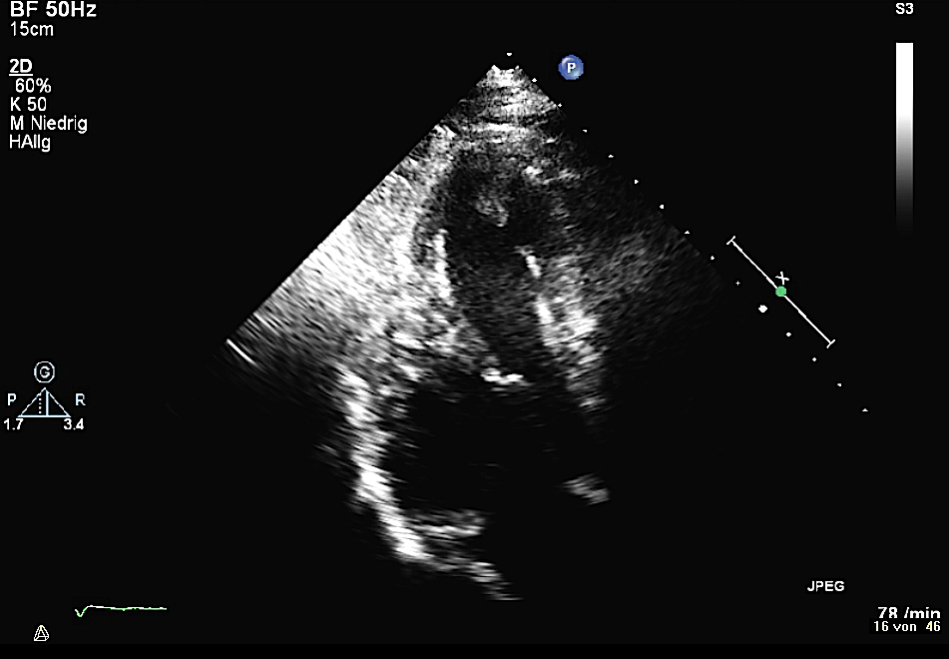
Transthoracic echocardiography; 4 chamber view reveals left ventricular thrombus.
